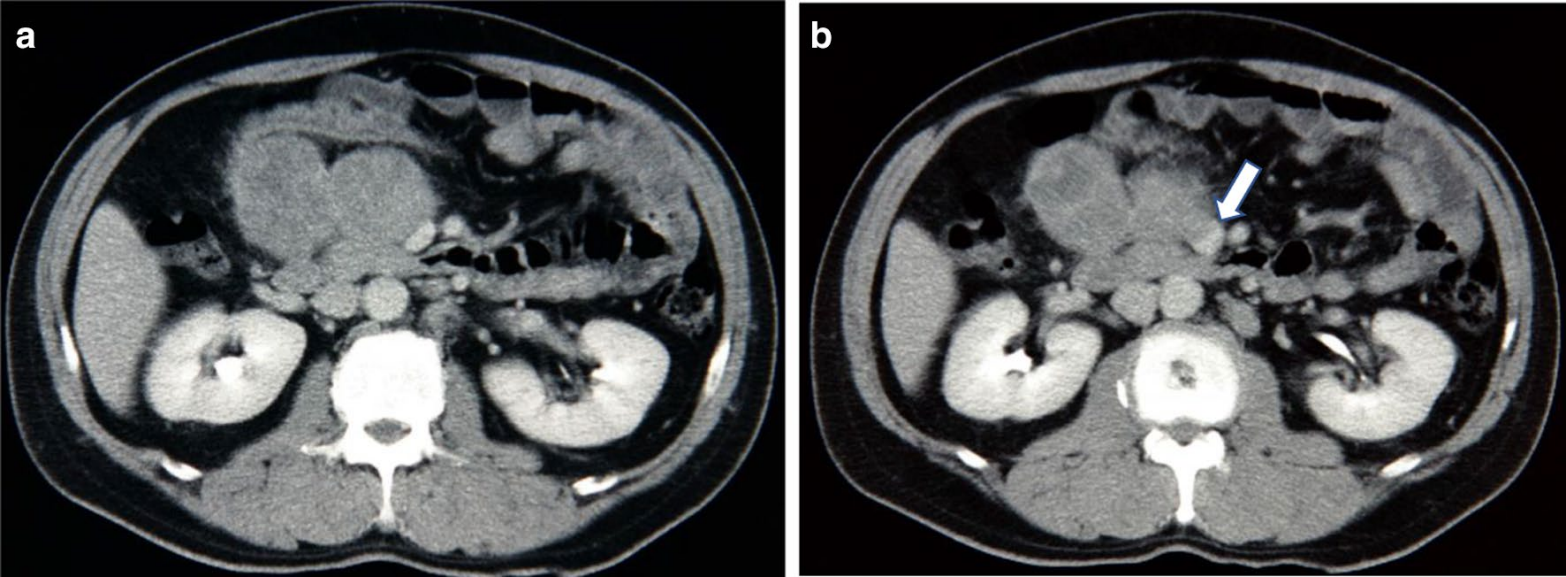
Abdominal computerized tomography (CT) showing two in-homogeneously enhanced round contiguous masses, 5 cm in size each, at the region of the pancreatic head (a), and the masses invading into the superior mesenteric vein (arrow) (b)
